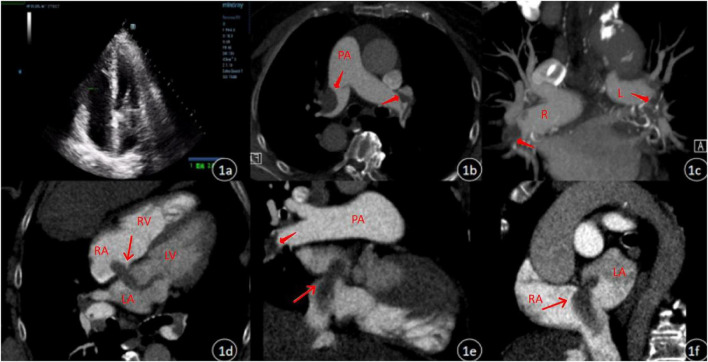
(a) Cardiac ultrasound demonstrates normal intracardiac structures and chamber dimensions; (b,c) CTA reveals thrombus in the left and right pulmonary arteries; (d–f) CTA shows an embedded, thin, earthworm-like thrombus visible in the foramen ovale region. RA, right atrium; LA, left atrium; RV, right ventricle; LV, left ventricle; PA, pulmonary artery.
